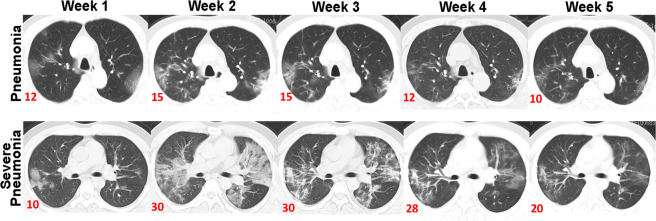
Representative temporal radiographic changes in one pneumonia and one severe case of COVID-19 patient at the indicated week. Numbers in red are HRCT scores.
